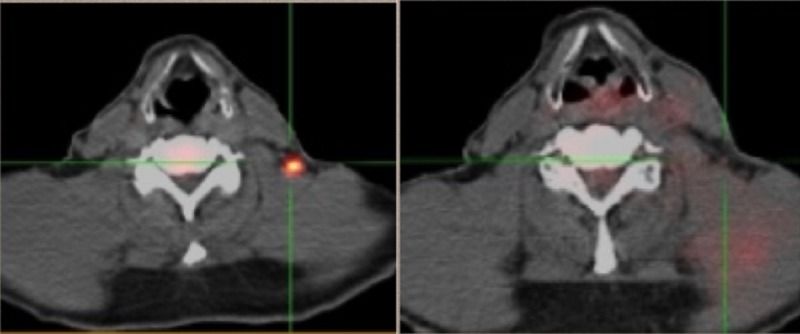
Pre/post PET/CT positron emission tomography-computed tomography: PET/CT
